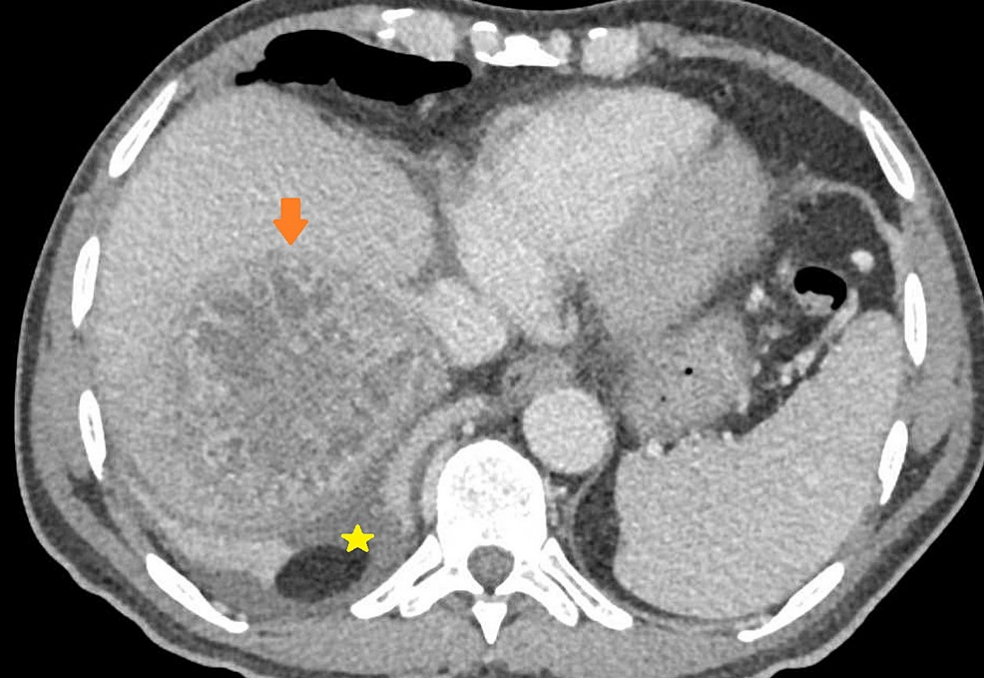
Contrast-enhanced computed tomography image of the upper abdomen in portal venous phase demonstrating a well-circumscribed collection (arrow) in segments 5 and 6 of the right lobe of liver with ragged edges and multiple internal septations. Note the localized perihepatic fluid collection (star) due to subcapsular extension of the abscess.
